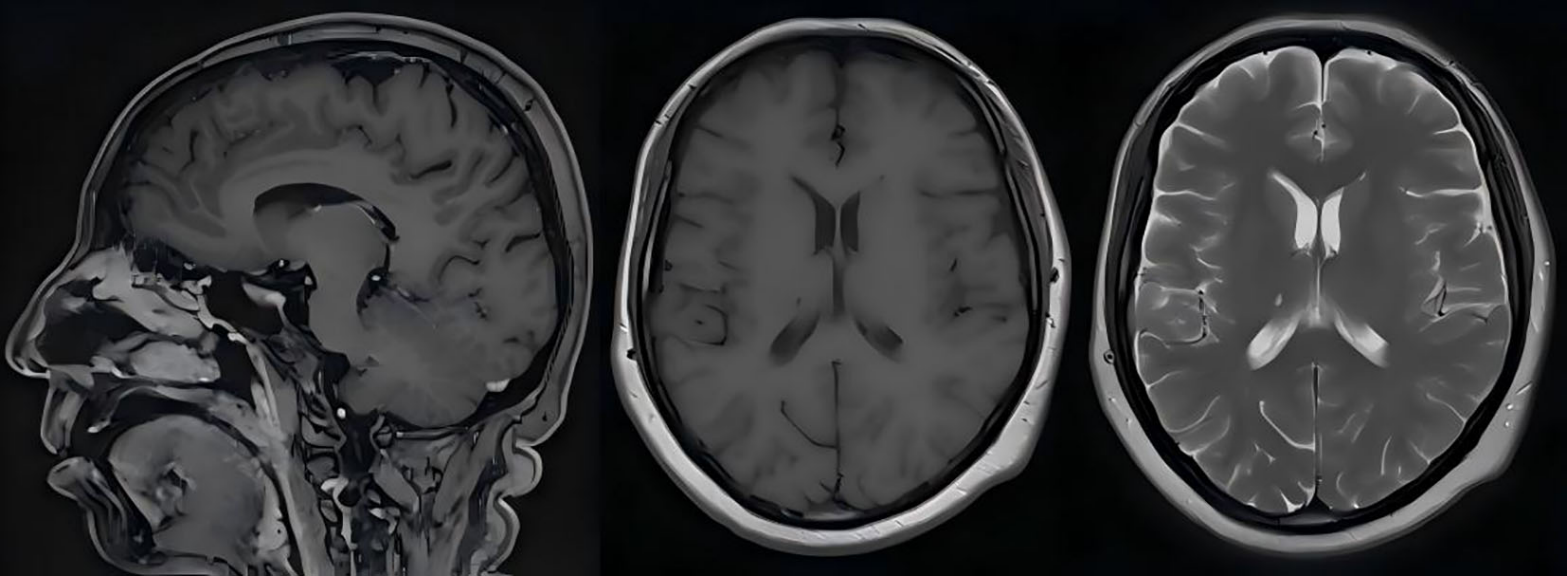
Despite T1, T2 magnetic resonance imaging with contrast of the head showed no signs of meningeal metastasis. On May 26, 2025, the patient suffered a seizure.
